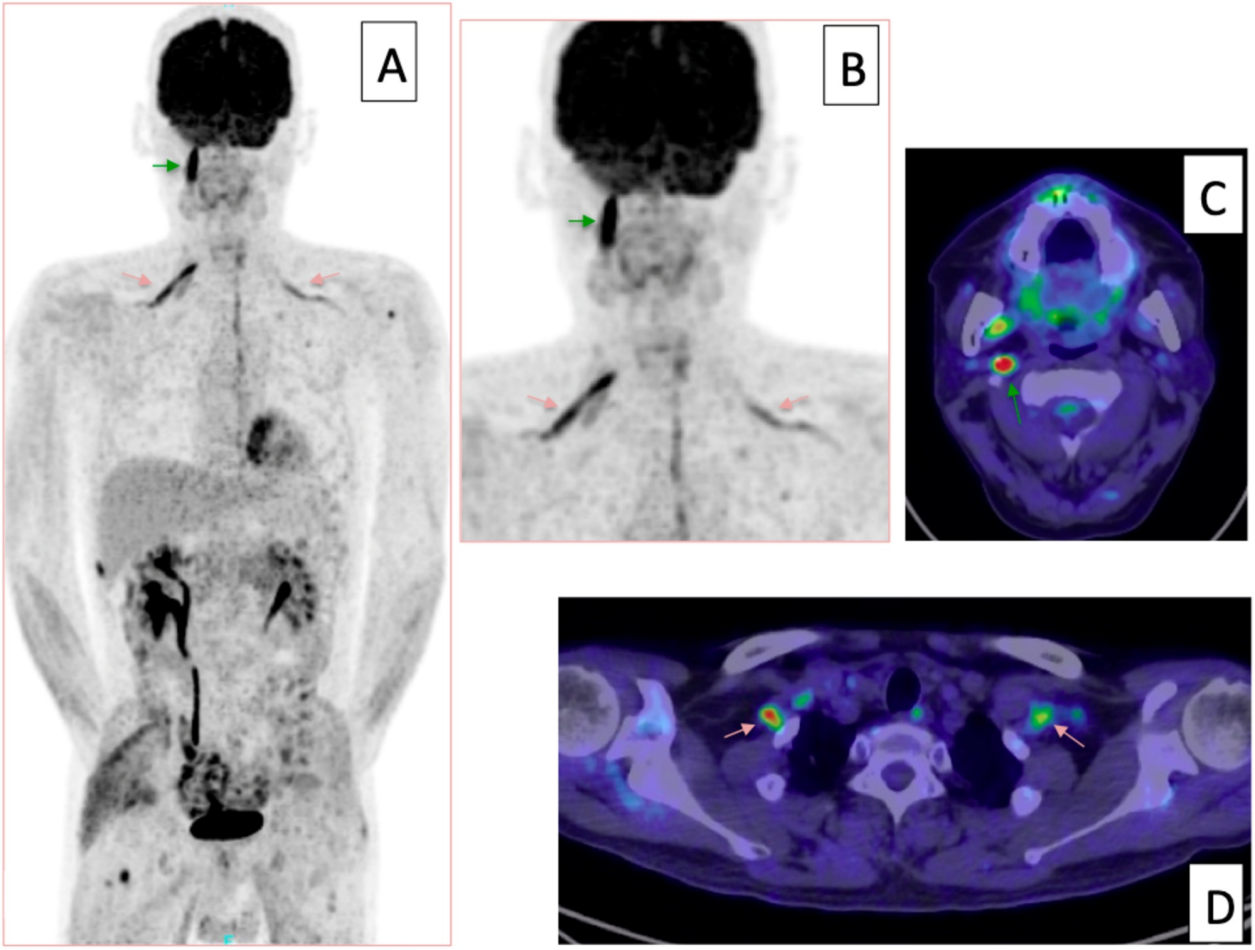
Subsequent fluorodeoxyglucose-positron emission tomography (FDG-PET) five weeks after craniospinal irradiation Fluorodeoxyglucose (FDG) accumulated in the right lower cranial nerve root (A, B, C, green arrows) and brachial plexus (A, B, D, red arrows). The radiation-induced esophagitis was almost totally relieved.
